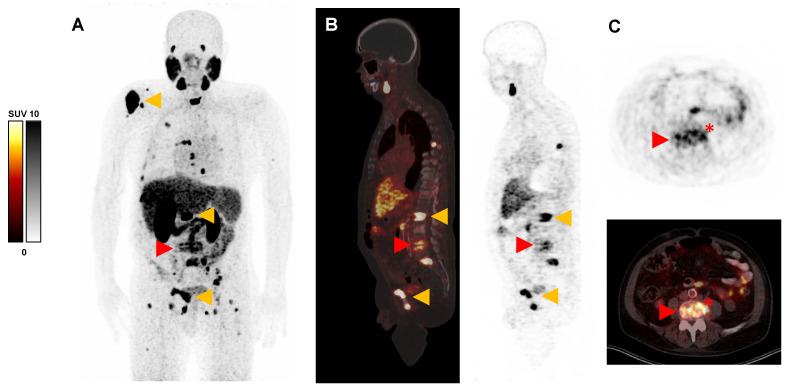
Prostate-specific membrane antigen (PSMA)-targeted positron emission tomography/computed tomography (PET/CT) scan with [^68^Ga]Ga-PSMA-11 (acquired 60 min post injection of 130 MBq) of a 71-year-old man with metastatic castration-resistant prostate cancer (mCRPC) referred for PSMA-targeted radioligand therapy. (A): Maximum intensity projection (MIP), (B): sagittal slices, and (C): transversal slices of [^68^Ga]Ga-PSMA-11 PET/CT, showing multiple intense PSMA-positive metastases (maximum standardized uptake value [SUVmax] up to 48.0, exemplary orange arrows) in the skeleton but also a moderate uptake (SUVmax 10.8) in the ground and upper plate of vertebral body L4 and L5, respectively (red arrow), also with involvement of the intervertebral space and surrounding soft tissue (red asterisk). Due to the distribution pattern, inflammatory etiology, i.e., spondylodiscitis, seemed more likely than additional metastasis, which could not be excluded at this point. Matching this differential diagnosis, the patient also reported newly developed pain in the lower back, and the inflammatory blood parameter, C-reactive protein (CRP), was highly elevated (61.8 mg/L). Neurological symptoms were not observed. Subsequently performed magnetic resonance imaging (MRI) confirmed the suspicion of active spondylodiscitis by showing low T1 and high T2 signal in disc space L4/5 and adjacent endplates, respectively. In contrast-enhanced T1 sequence vertebral endplates and paravertebral soft tissue revealed substantial enhancement. After the microbiological identification of streptococcus gallolyticus and subsequent appropriate antibiotic treatment, both pain and blood inflammation levels regressed. Spondylodiscitis mainly occurs in the elderly and is usually diagnosed on the basis of clinical, laboratory, and microbiological examinations, as well as imaging techniques such as CT, MRI, and PET with ^18^F-Fluorodeoxyglucose ([^18^F]FDG) [1,2,3,4,5]. In contrast, the finding of spondylodiscitis is rarely described on a PSMA PET/CT [6,7]. However, it is known that inflammatory processes may be related to the increased uptake of PSMA ligands, in line with our observed case of spondylodiscitis [8,9]. This interesting image should draw attention to spondylodiscitis as a differential diagnosis of PSMA-positive findings in the spine, especially when the intervertebral space and soft tissue are involved.
